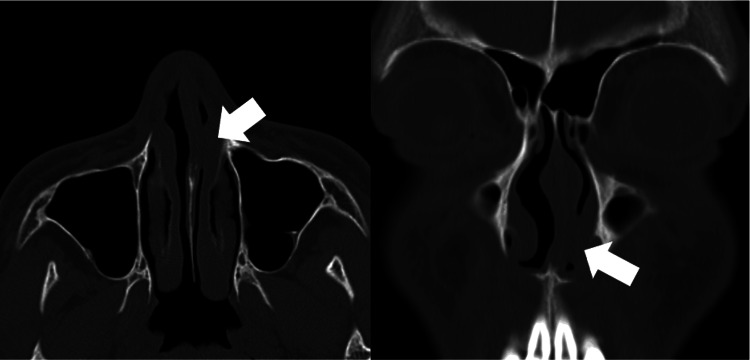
Sinus computed tomography images Sinus computed tomography showed contact between the nasal septum and the left inferior turbinate (white arrow).
